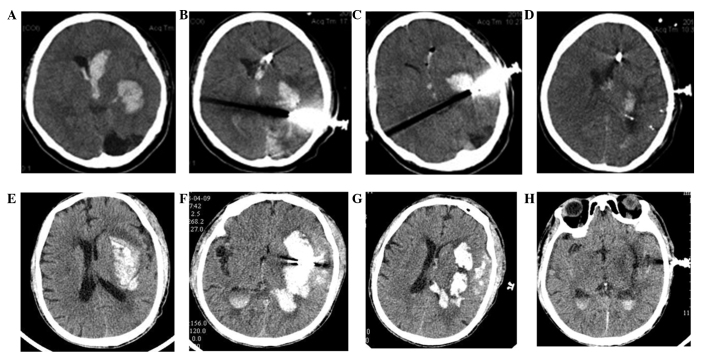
Brain CT film results of patients treated with (A–D) minimally invasive evacuation of hematoma 3 h after the onset, and (E–H) minimally invasive evacuation of hematoma and subsequent mild hypothermia 2 h after the onset. Brain CT film was obtained at (A) 3 h after the onset; (B) 1 day after surgery; (C) 3 days after surgery; (D) 7 days after surgery; (E) 2 h after the onset; (F) 1 day after surgery; (G) 3 days after surgery; and (H) 7 days after surgery. CT, computed tomography.
